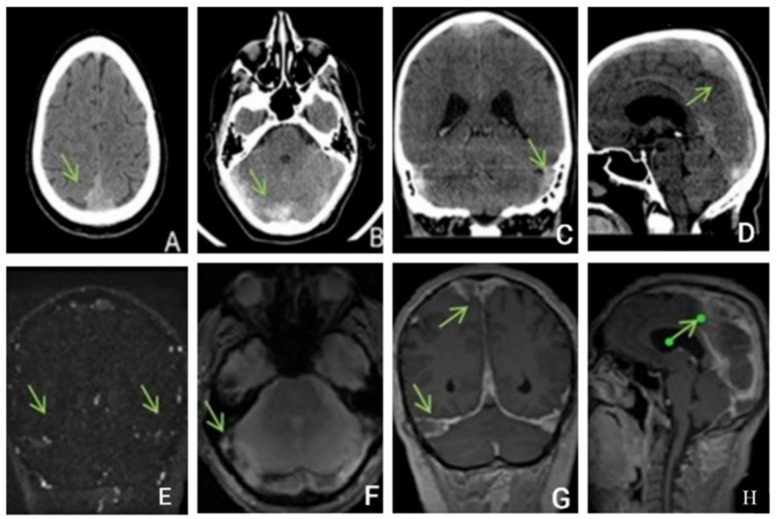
Unenhanced CT: (A,B)—axial, (C)—coronal, (D)—sagittal exhibits hyperdensity of the venous sinuses (sagittal superior and inferior, right sinus, transverse and sigmoid sinuses). (E–H) MRI, (E)—TOF (time of fligt angiography) venous coronal; (F)—T2*hemo axial, (G)—T1 coronal after i.v. contrast, (H)—T1 sagittal after i.v. contrast, expressed extensive thrombosis of all veins.
